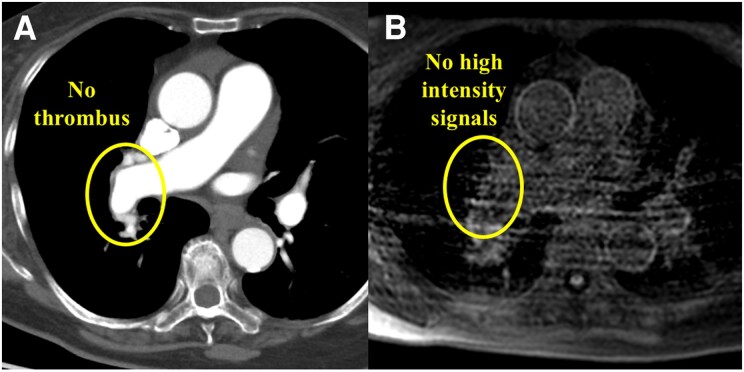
CTPA and MRDTI images after 6 months of anticoagulation therapy. (*A*) No thrombi could be seen in the proximal PA on CTPA. (*B*) The MRDTI image shows no high-intensity signal in the area corresponding to the thrombi detected by CTPA at the patient’s initial referral. CTPA, computed tomography pulmonary angiography; MRDTI, magnetic resonance direct thrombus imaging; PA, pulmonary artery.
